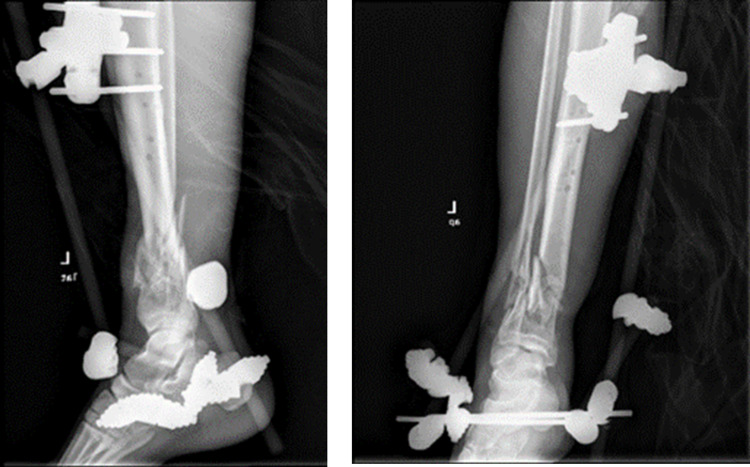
Post-operative x-rays revealing bone loss of distal tibia.
